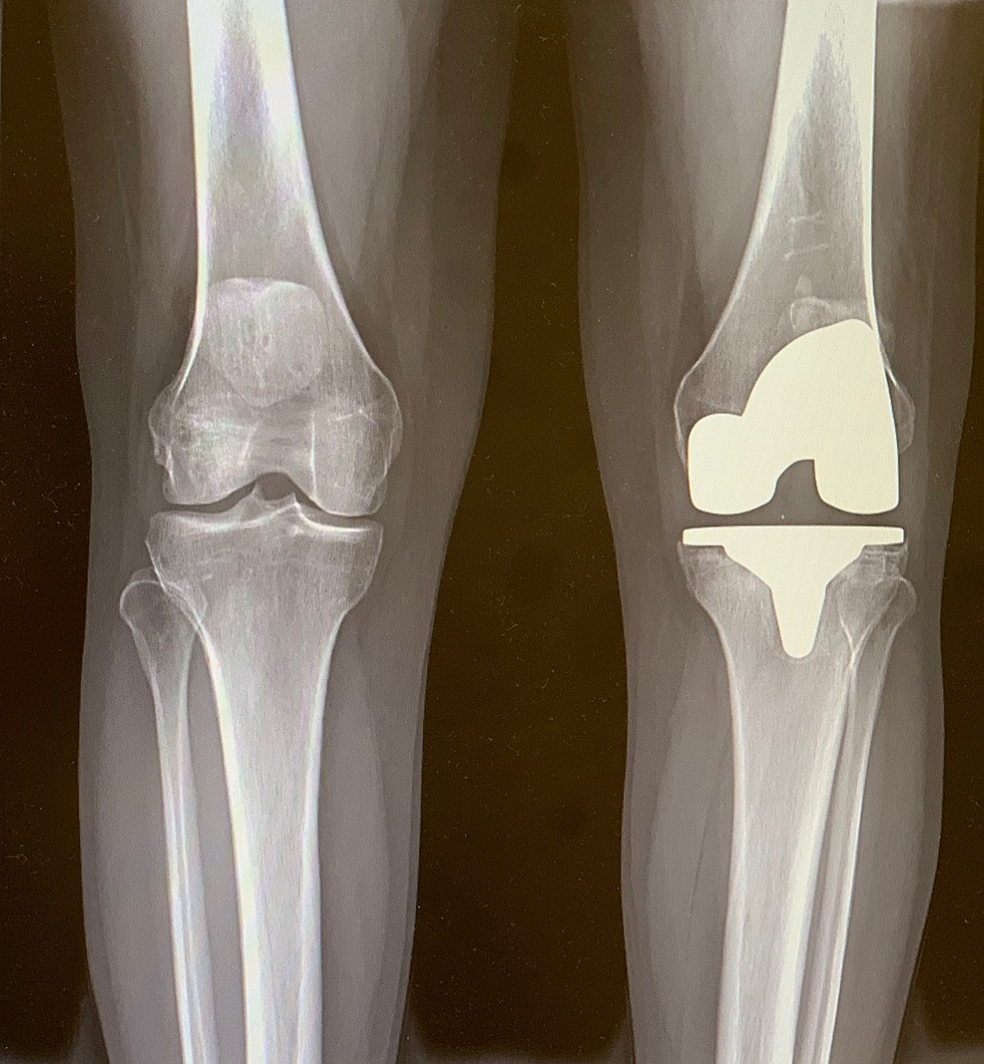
Preoperative standing AP radiograph of the bilateral knees demonstrating no obvious signs of aseptic loosening of the tibial baseplate. AP: anteroposterior
